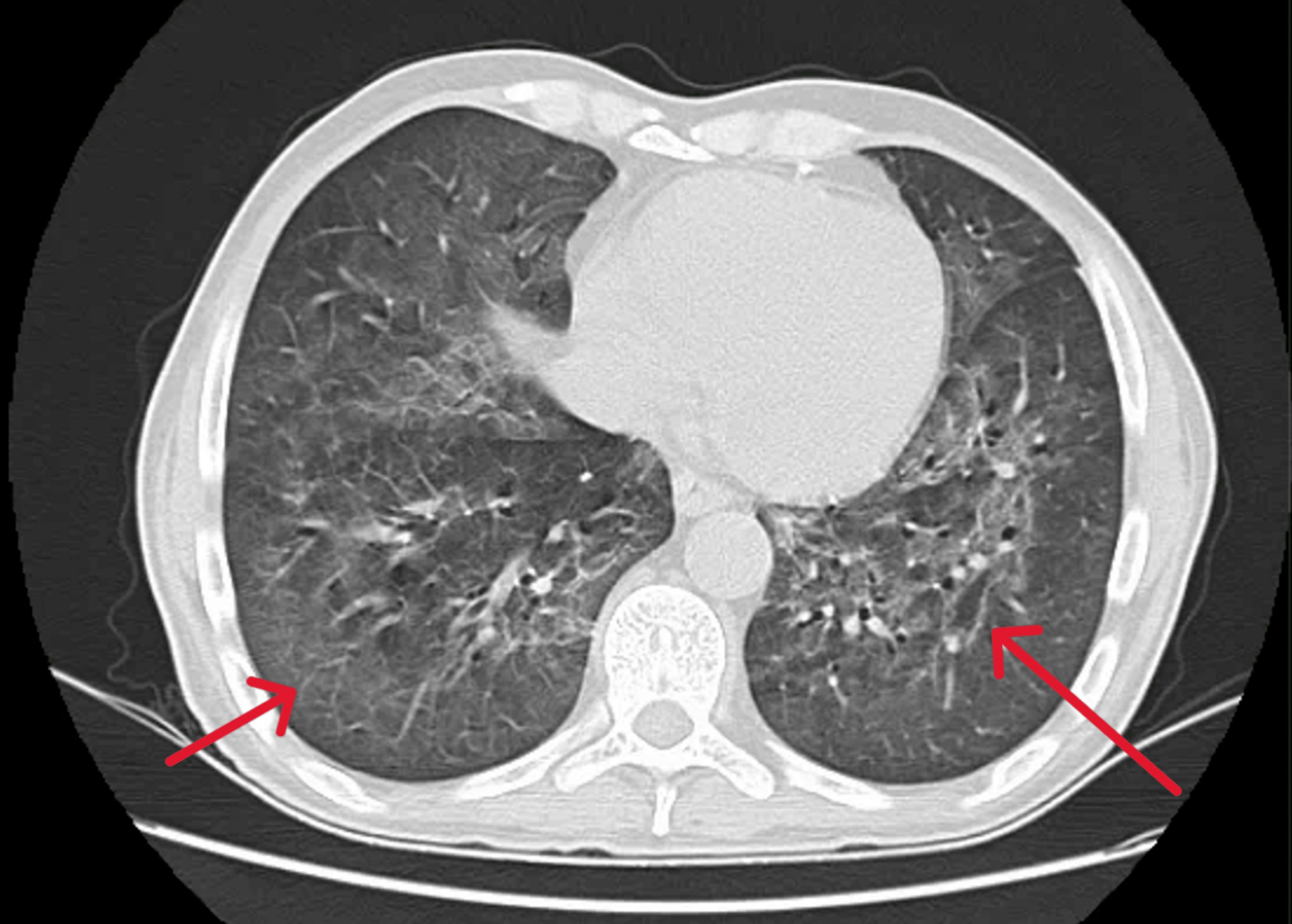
High-resolution CT scan of the lungs showing the bilateral ground-glass opacities (red arrows) CT: computed tomography
